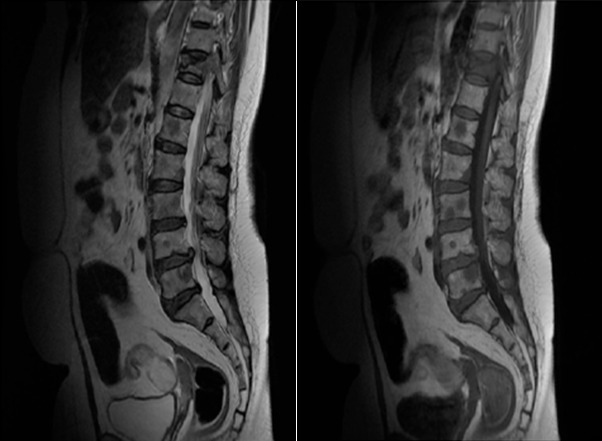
Radiological aspects of bone metastases on MRI images in T1 and T2 sequences
